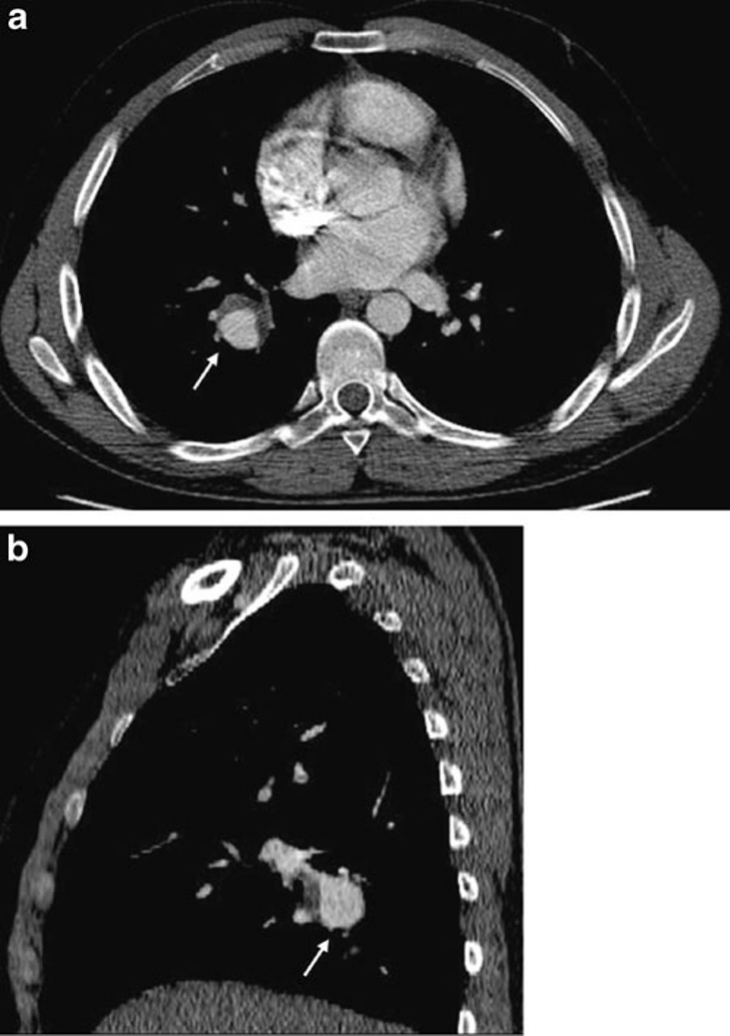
a, b A 23-year-old male with Behçet's disease. Axial (a), sagittal reformatted (b) contrast-enhanced CT images show partially thrombosed right pulmonary artery aneurysm (*arrow*)
